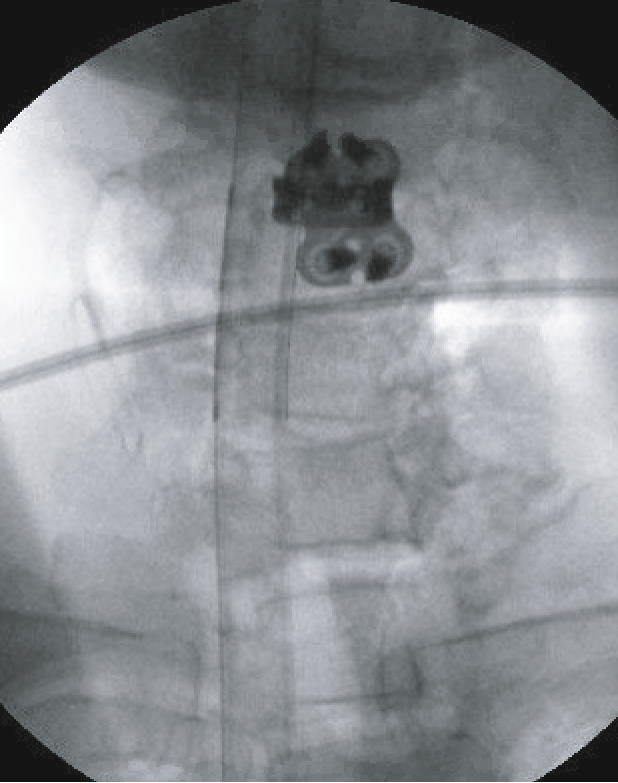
Anterior-posterior intraoperative radiograph of the cervical spine, status post-C4 and C5 anterior cervical discectomy and fusion.
